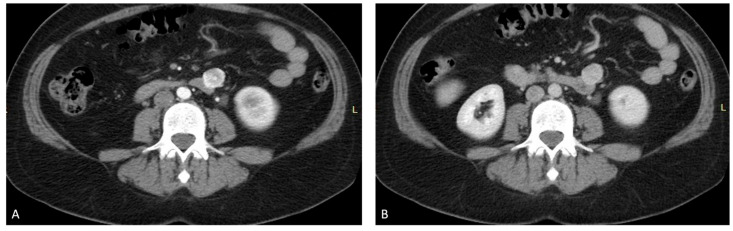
Abdominal CT with contrast: (A) arterial time; and (B) venous time. Welldefined tumor with necrotic core.
